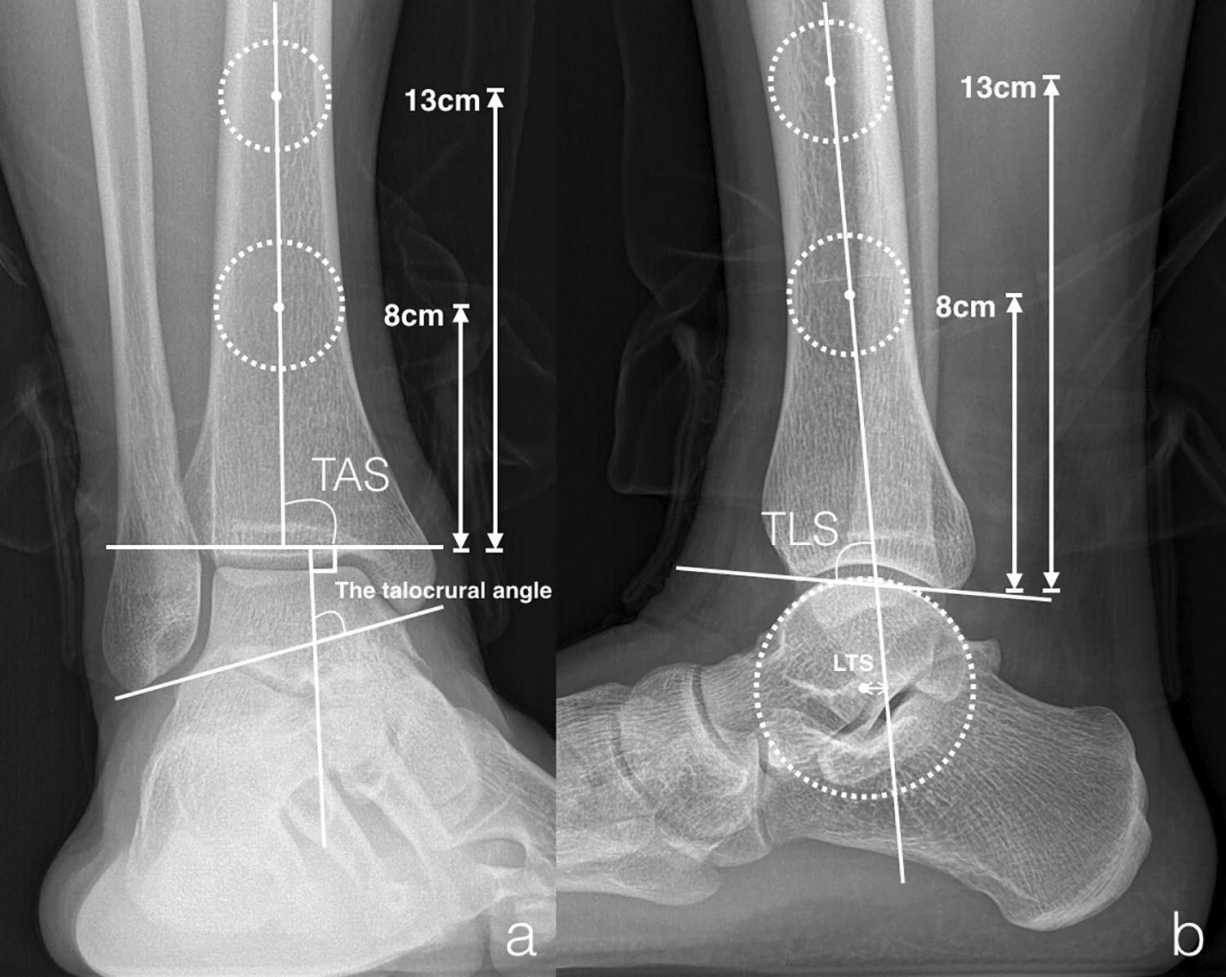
Radiographic measurement. (a) TAS, tibial anterior surface; (b) TLS, tibial lateral surface; LTS, lateral talar station.
